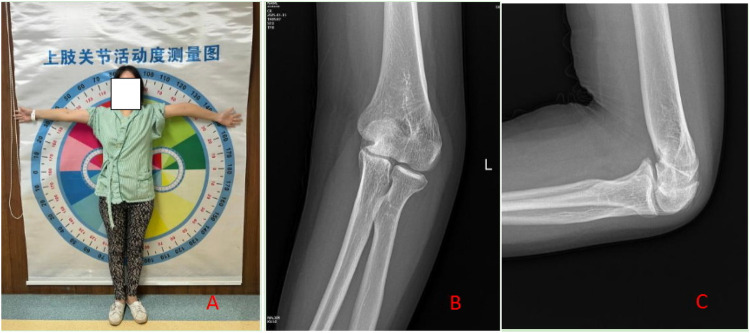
(A–C) preoperative photograph and radiographs showed cubitus varus deformity of left elbow.
